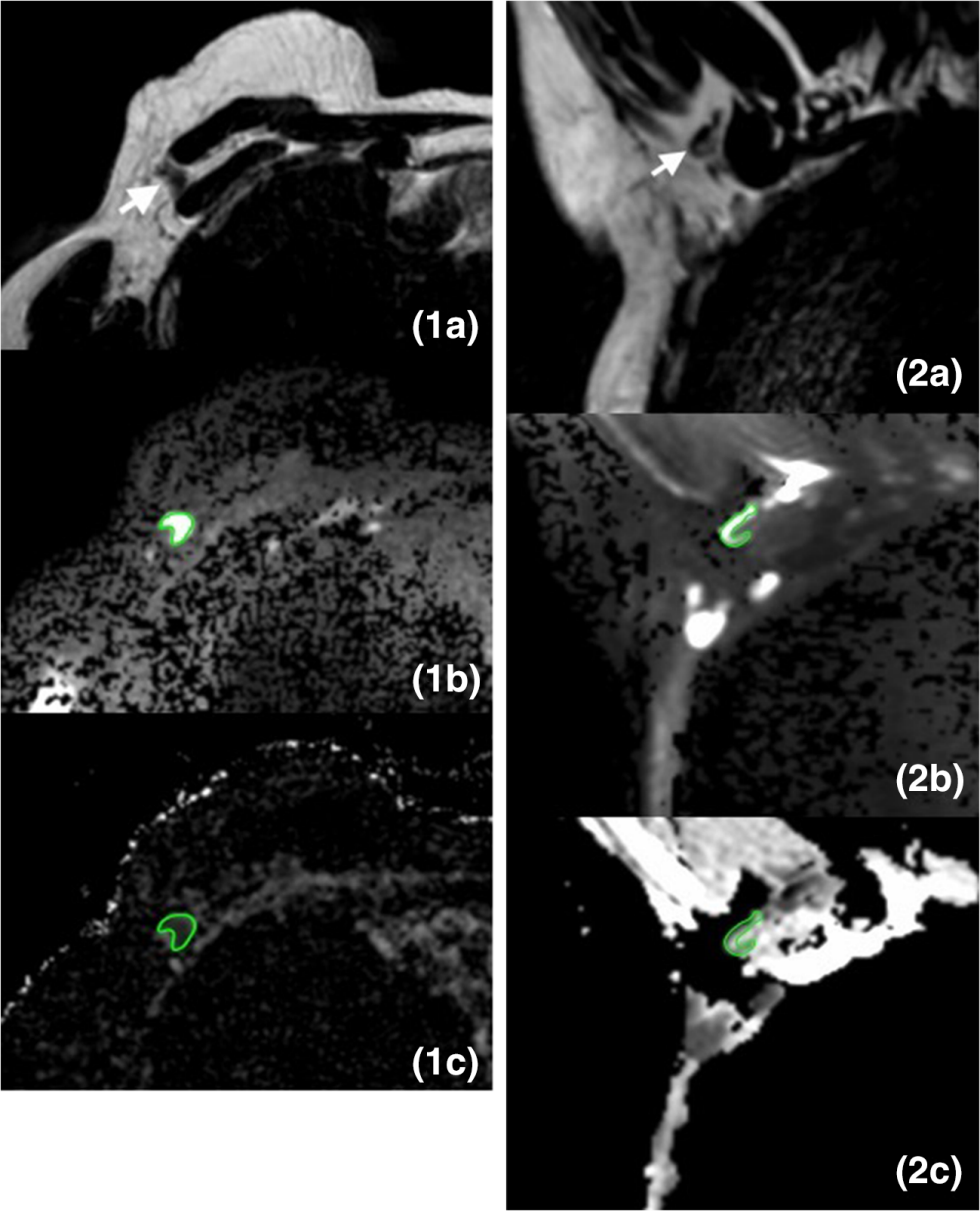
Images of a 27-mm large invasive ductal carcinoma in the right breast of a 64-year-old female patient, which was treated with mastectomy and ALND (pT2N1). The white arrow points to an axillary lymph node. The green delineation shows an axillary lymph node. 1a Axial T2W breast MR image shows the axillary lymph node with the longest axis. 1b Axial DW breast MR image (*b*-value = 800 s/mm^2^) shows the same axillary lymph node with relatively high signal intensity. 1c Axial ADC map of breast MRI shows corresponding lymph node with relatively low signal intensity with an ADC value of 0.646 × 10^−3^ mm^2^/s. 2a Coronal T2W dedicated axillary MR image shows the axillary lymph node with the longest axis. 2b Coronal DW dedicated axillary MR image (*b*-value = 800 s/mm^2^) shows the same lymph node with relatively high signal intensity. 2c Coronal ADC map of dedicated axillary MRI shows corresponding lymph node with relatively low signal intensity with an ADC value of 0.837 × 10^−3^ mm^2^/s
